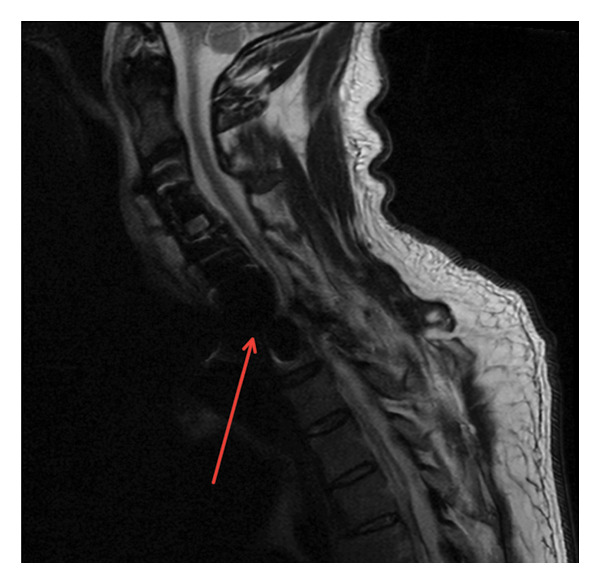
Cervical and thoracic spine MRI showing a partially imaged, extensive posterior epidural collection consistent with abscess formation extending from C7 through the thoracic spine (red arrow). Additional findings include prevertebral phlegmon and suspected abscess from C3 to C5, as well as contrast enhancement adjacent to the prior C3–C5 anterior cervical graft, suggestive of granulation tissue or infectious/inflammatory changes. Multilevel spondylotic changes are also noted.
